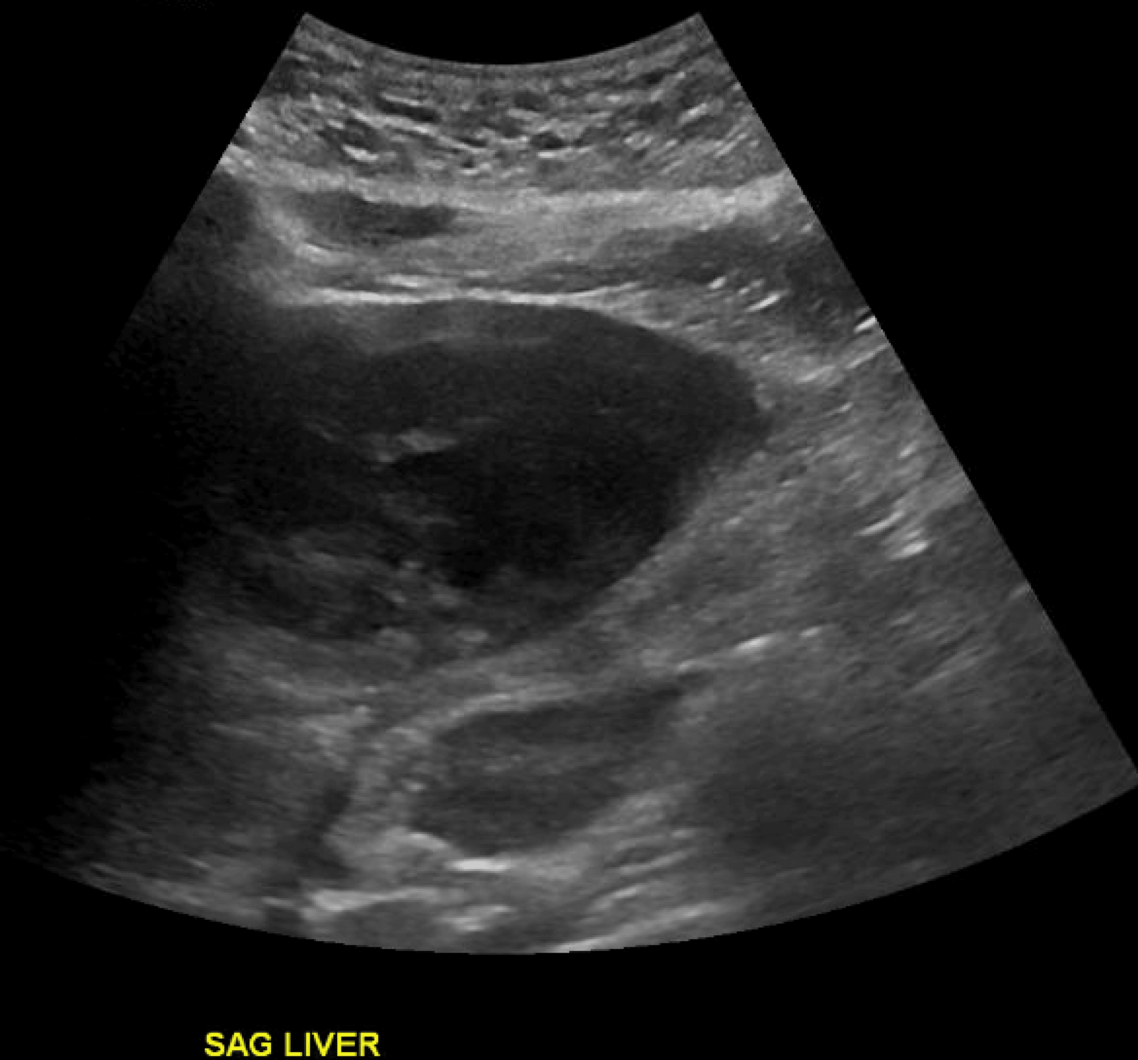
Ultrasound (US) of the right upper quadrant. Ultrasound (US) of the right upper quadrant obtained three days after presentation. Imaging demonstrates an ill-defined, heterogenously large, hypoechoic avascular lesion in the liver with possible fluid or necrosis present in the center. These findings are similar to characteristics appreciated on the initial US obtained on the day of presentation.
